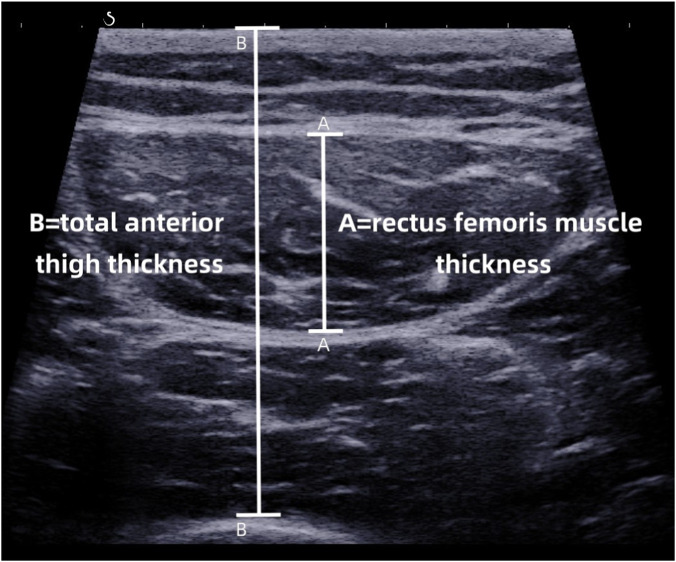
Ultrasound scan with measurements of the dominant thigh. A = rectus femoris muscle thickness; B = total anterior thigh thickness. Rectus femoris contraction index (RFci) = A/B.
